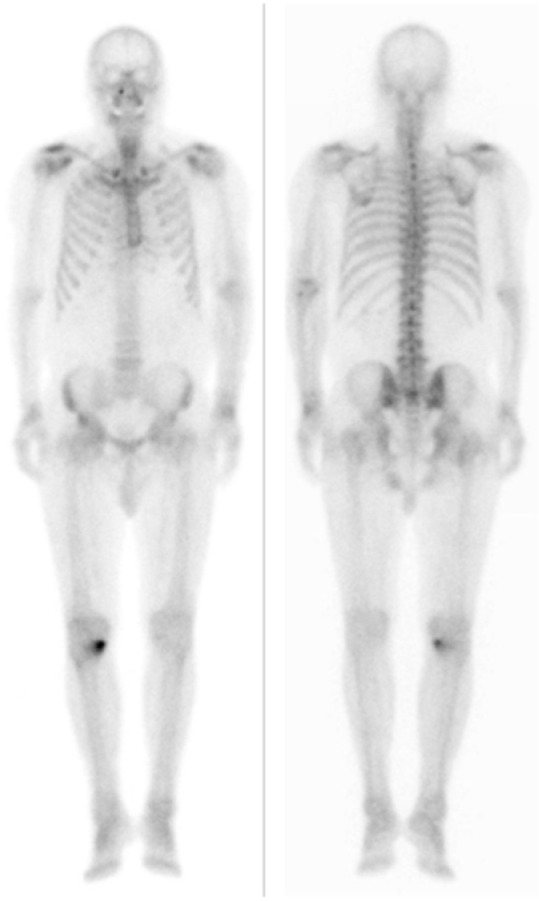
Bone scintigraphy with technetium-99m hydroxymethylene diphophonate (HDP) shows no cardiac accumulation.
